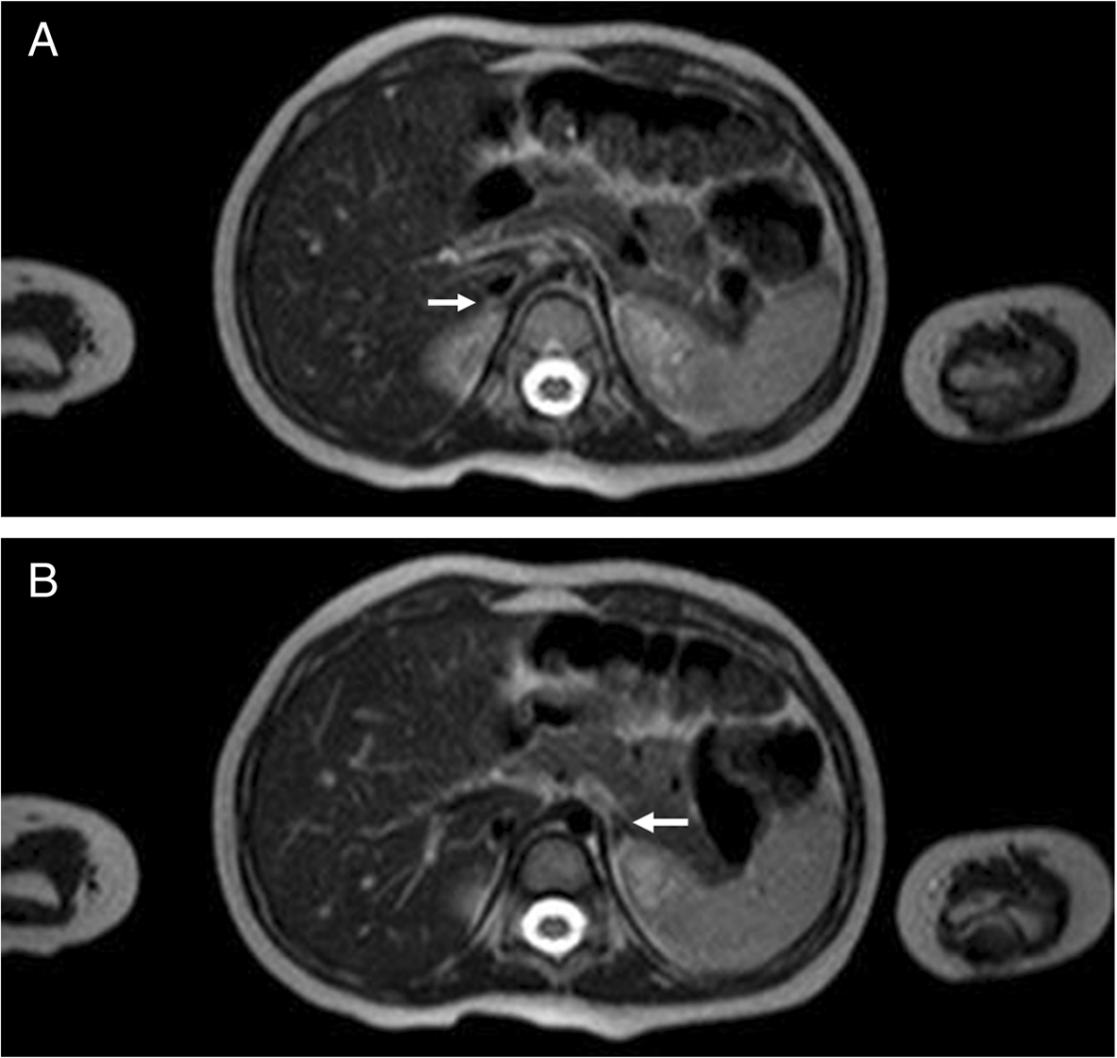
MRI scan of the bilateral adrenal glands revealing agenesis of the right (A) and hypoplasia of the left (B) adrenal glands.
